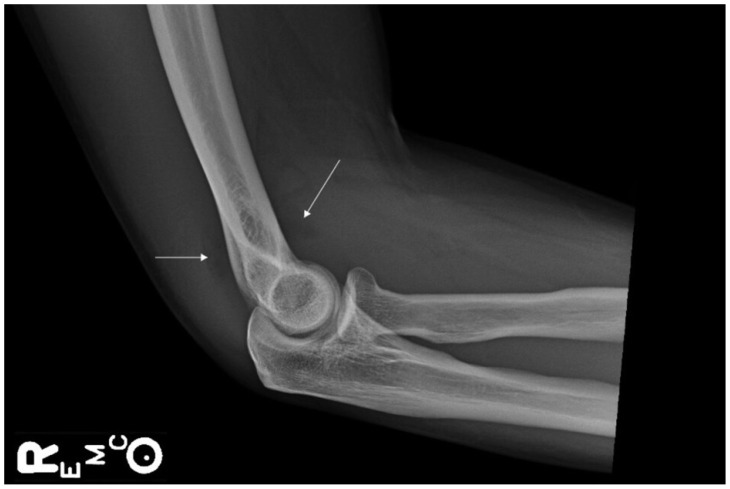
Lateral radiograph of right arm. Arrows identify displacement of the anterior and posterior fat pads consistent with a joint effusion.
